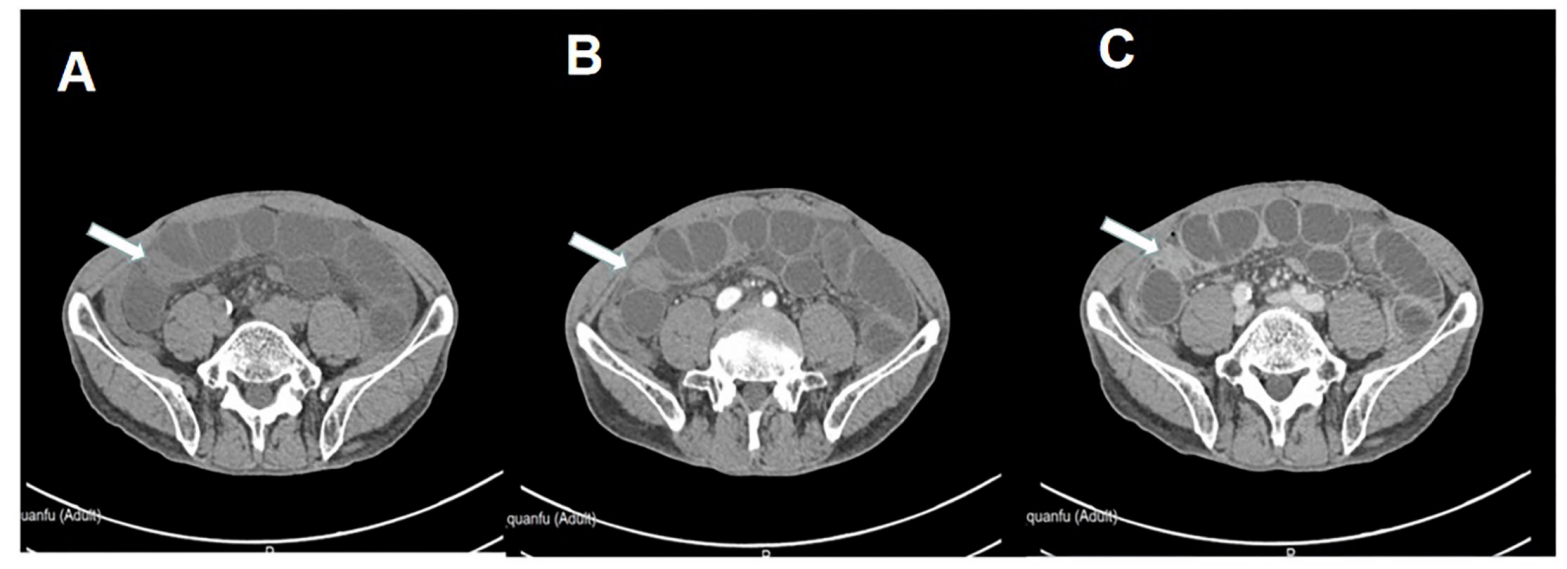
Abdominal enhanced CT suggests that incomplete small bowel obstruction should be considered, the arrow points to the site of the obstruction. (A) Plain scan. (B) Arterial phase. (C) Venous phase.
